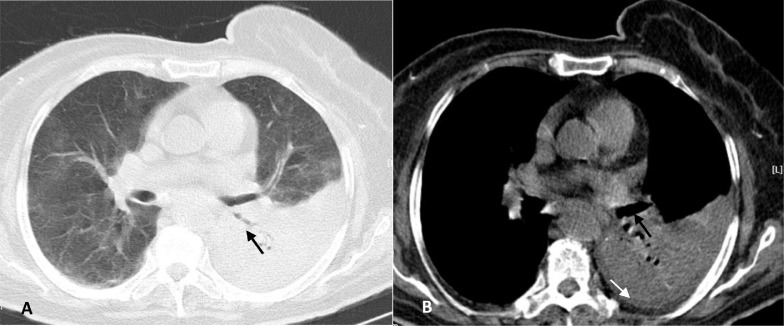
Noncontrast CT scan of lung (A: lung window, B: mediastinal window) shows narrowing and obliteration of the LLL bronchus (marked with black arrow) which resulted in LLL collapse/consolidation. Subtle left side pleural effusion is also noted (marked with white arrow).
